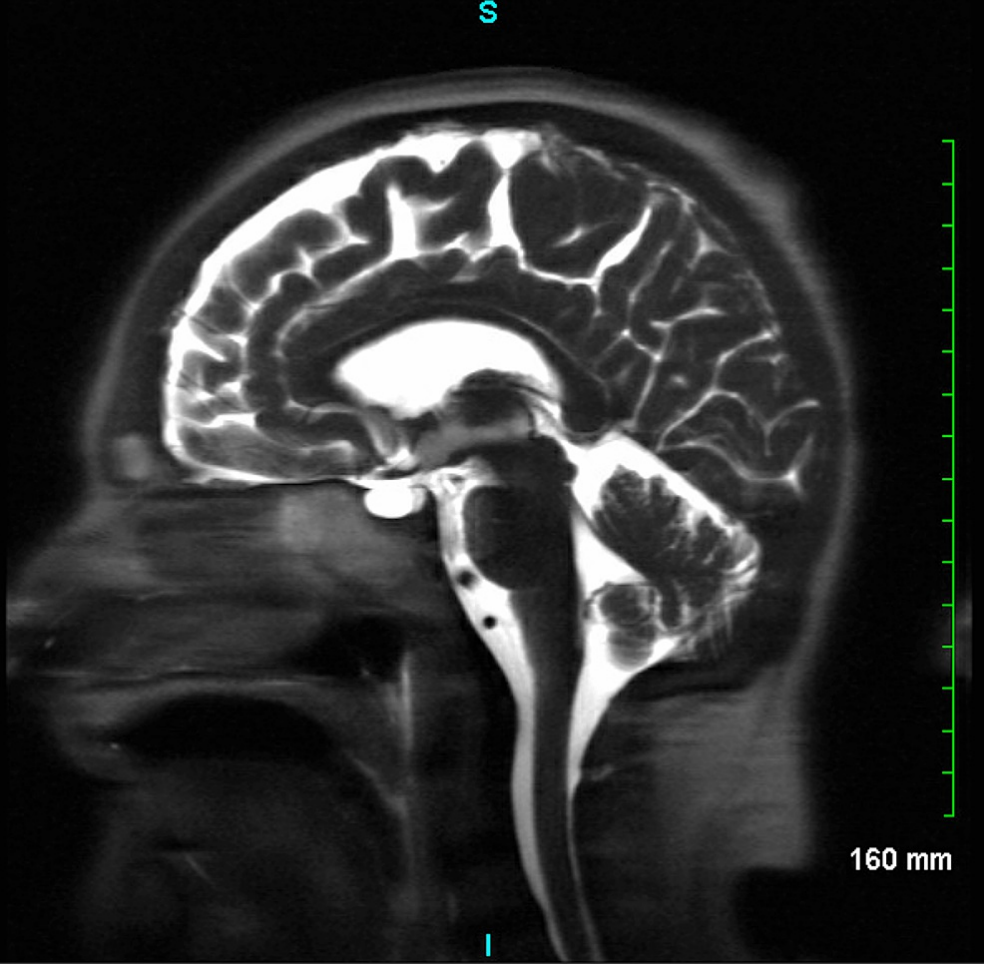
Brain MRI was negative for any findings of acute intracranial processes. White matter abnormalities were consistent with small-vessel ischemic changes and remote bilateral lacunar infarcts in the cerebellum, none of which were pertaining to her symptoms of AIDPR. MRI: magnetic resonance imaging; AIDPR: acute inflammatory demyelinating polyradiculoneuropathy
